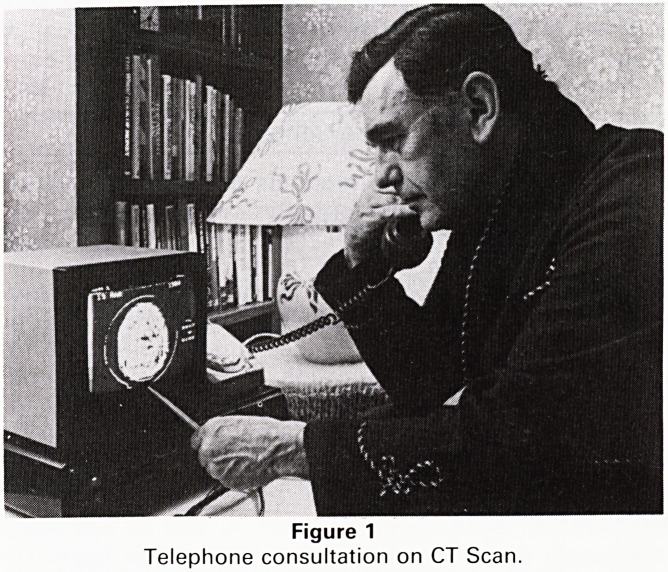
# The Imtran Revolution, Telephone Transmission of X-Rays and Scans
